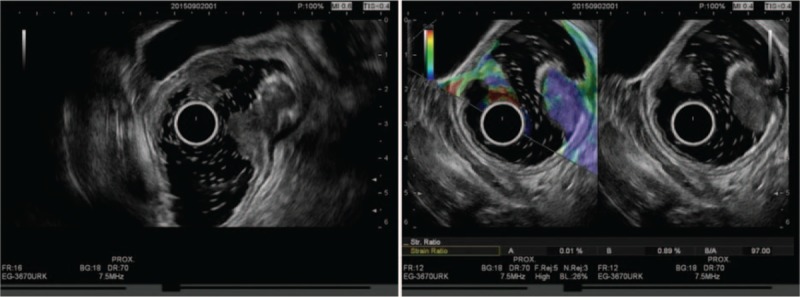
Endoscopic ultrasonography showed a low-echo area in the muscularis mucosae layer and no invasion into the submucosal layer, without enlarged celiac lymph nodes.
